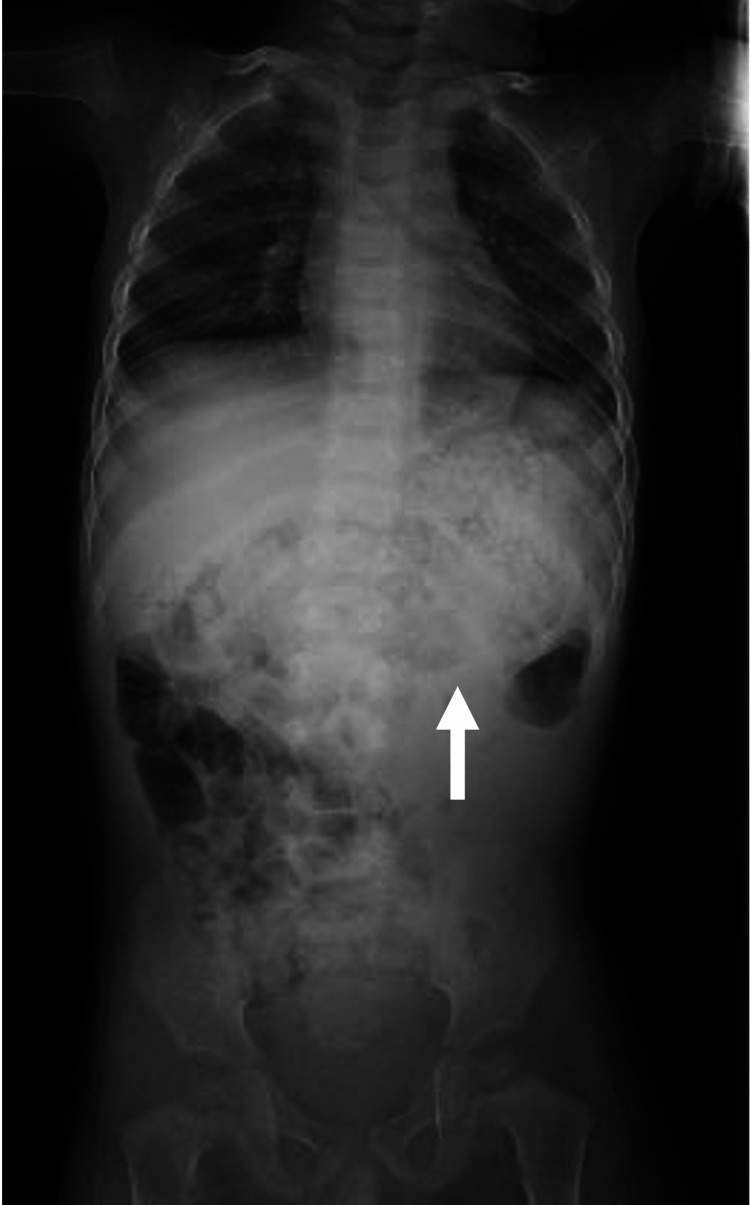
Abdominal X-ray of the patient at age 1 year and 6 months The white arrow shows the dilated stomach
